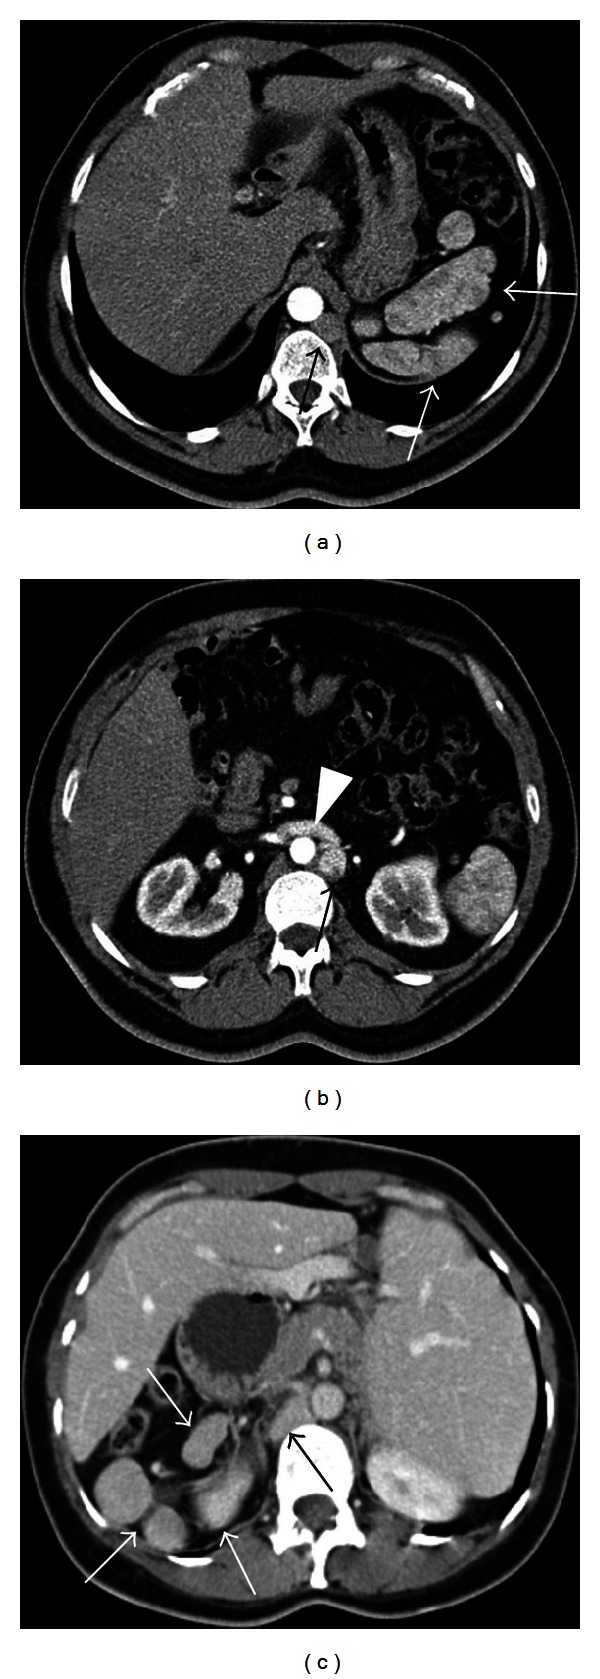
Heterotaxy syndrome in 48-year-old male (a), (b) and 49-year-old female patients (c). Axial contrast enhanced CT images (a), (b) show polysplenia (white arrows) at left upper quadrant which have heterogenous enhancement pattern on arterial phase as splenic tissue, azygos continuation (black arrow) of the inferior vena cava, and right renal vein draining into the azygos vein (arrowhead). Intrahepatic portion of the inferior vena cava is absent. Axial contrast enhanced CT image (c) shows, polysplenia at right upper quadrant (white arrows), absence of spleen at its normal location, liver located at midline related to situs ambiguous, and dilated azygos vein (black arrow).
